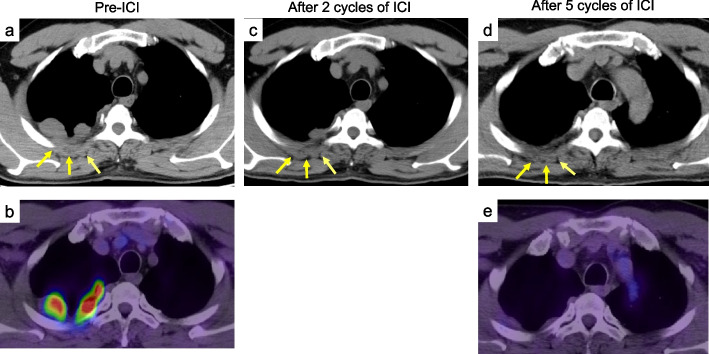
Dramatic preoperative effects of immune checkpoint inhibitors (ICI). Chest CT and ^18F−^FDG-PET findings showed pleural thickening of the dorsal right upper lobe and multiple nodules, including a nodule with a maximum diameter of 34 mm, which was suspected to have invaded the chest wall (cT4) (arrows) (a), which showed an abnormal SUV uptake value of 19.9 (b). After two cycles of ICI, the tumor has shrunk, and the part invading the chest wall has become less distinct (c). After five cycles of ICI, further tumor regression (d) and abnormal SUV uptake (e) were not observed
